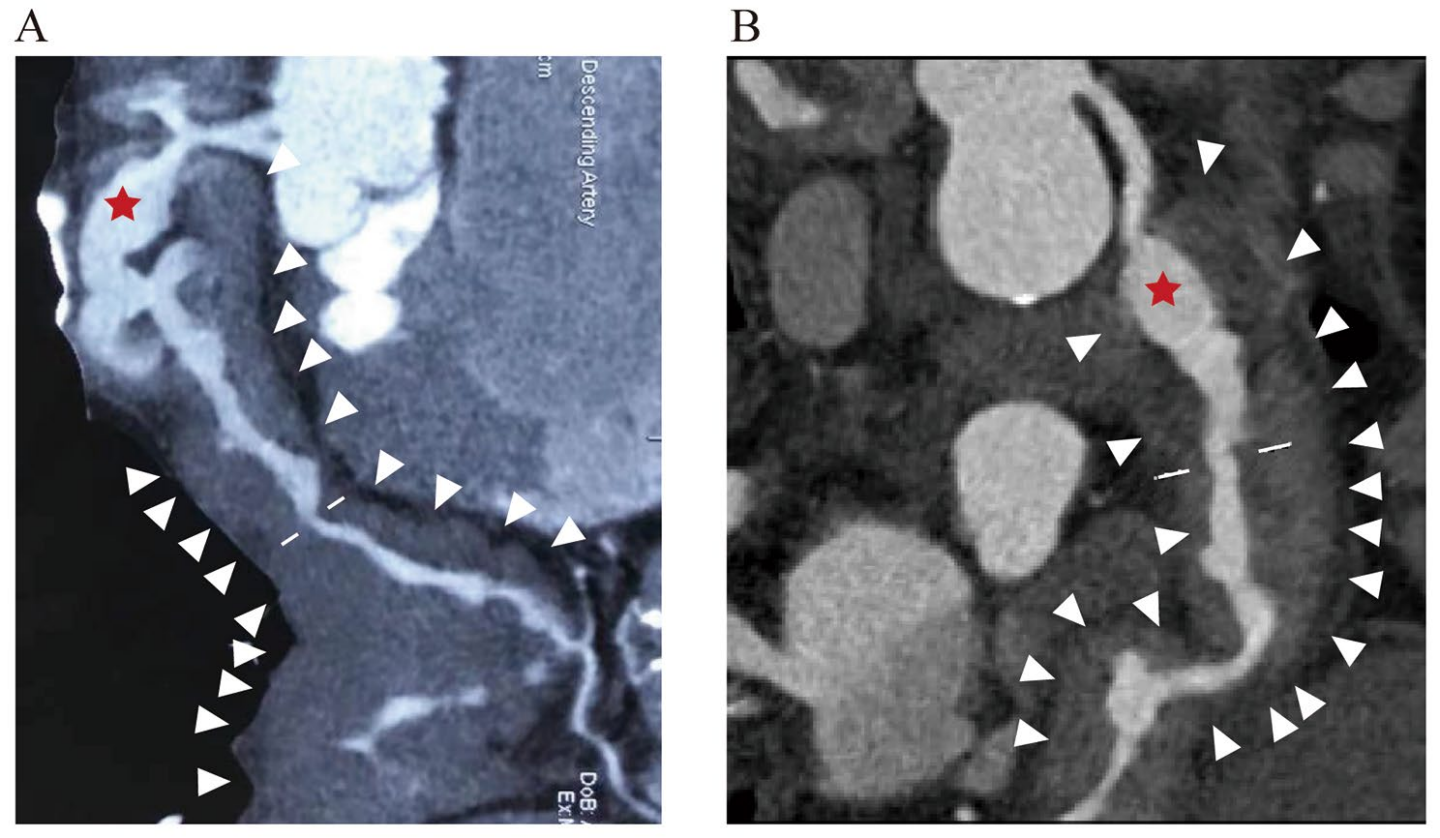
(A) Curved multiplanar reformatted coronary artery CTA demonstrate multifocal aneurysmal dilatation (red asterisk) and mild-to-moderate stenosis (line) of the LAD with diffuse mural thickening (triangle). Repeated contrast-enhanced CT scan with curved multiplanar reformatted (B) revealed radiological remission with reduction of the diffuse mural thickening of LAD after treatment with glucocorticoid
